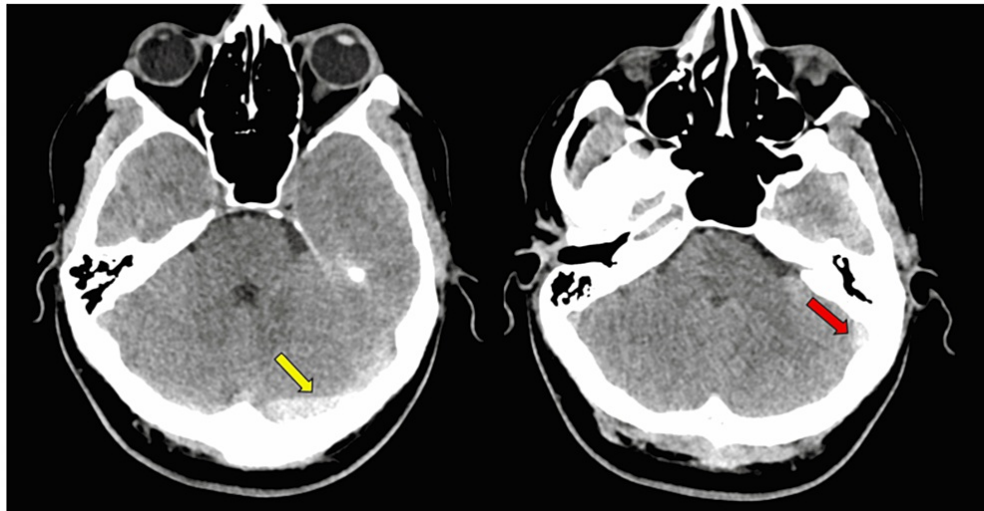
Axial head CT scan showing hyperdensity suggesting cerebral venous thrombosis within the left transverse sinus (yellow arrow) and beginning of the sigmoid sinus (red arrow).
